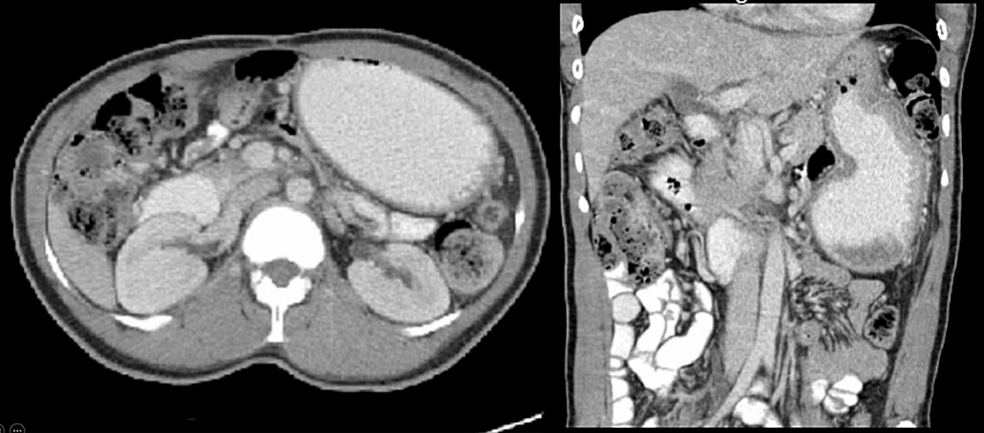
Abdominal CT showing dilated stomach with collapsed proximal duodenum suggesting gastric outlet obstruction.
